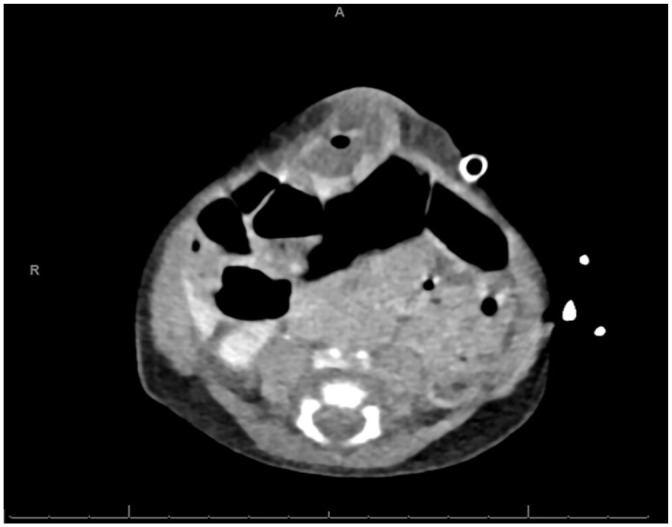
Axial view of abdominal computed tomography showing hypodense regions in the anterior abdomen and a small air pocket within those regions.
